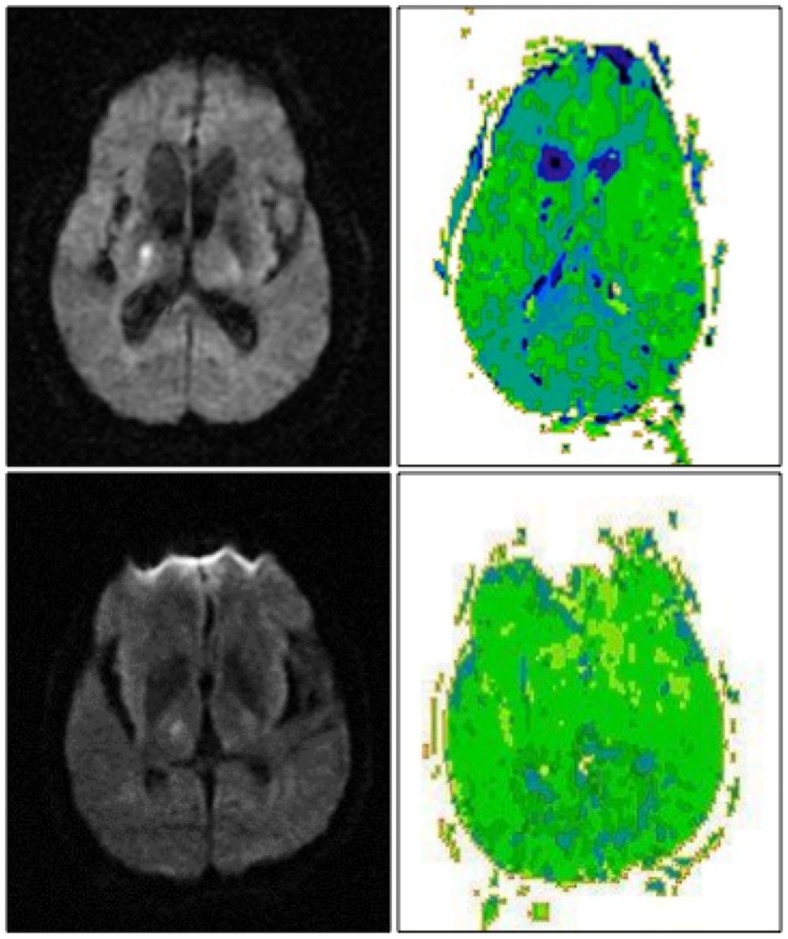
Right thalamic infarct with cortical hypoperfusion and associated neglect (top) and without cortical hypoperfusion and no associated neglect (bottom). DWI scans are shown on the left, PWI scans are shown on the right. Scans are in radiological convention (right hemisphere on left). Blue/darker green areas are hypoperfused.
